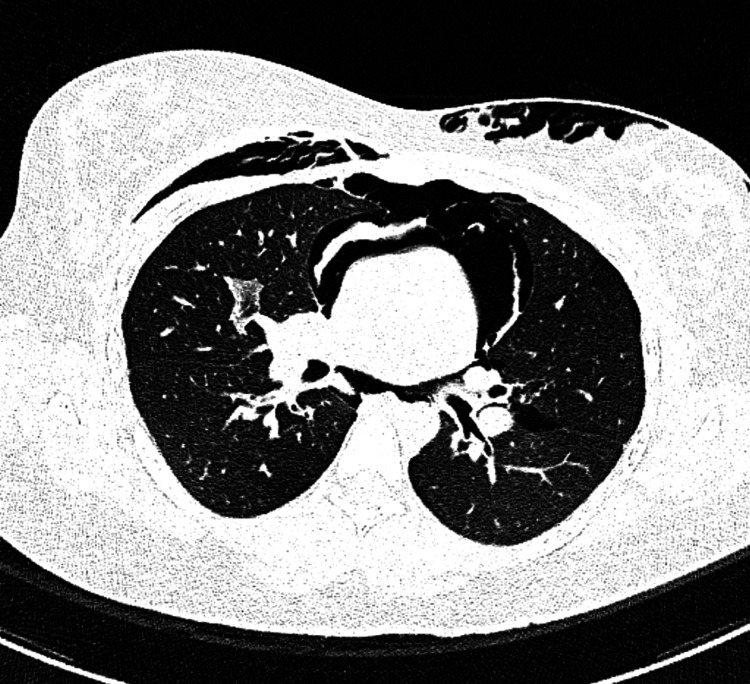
High-resolution computed tomography of the chest showing pneumomediastinum and extensive subcutaneous emphysema
